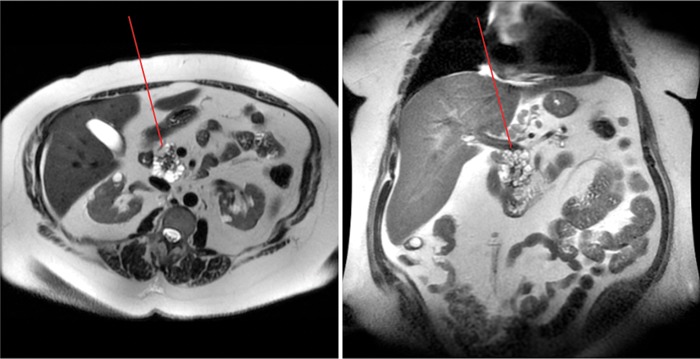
MRI of the abdomen of the 53-year-old woman 1 month before surgery reveals a cystic mass in the head of the pancreas (arrow) with distal pancreatic atrophy. Periportal and peripancreatic lymphadenopathy is appreciated.
